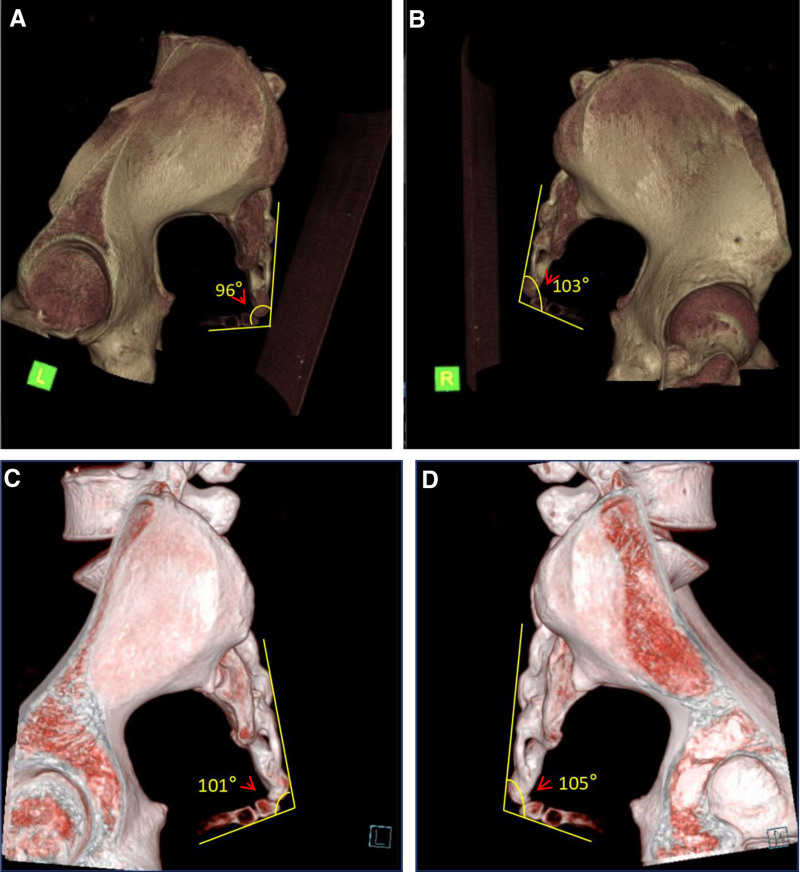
Pretreatment and posttreatment CT 3D reconstruction. (A) Pretreatment CT 3D reconstruction (February 13, 2025); (B) posttreatment CT 3D reconstruction (March 20, 2025). CT = computed tomography.
